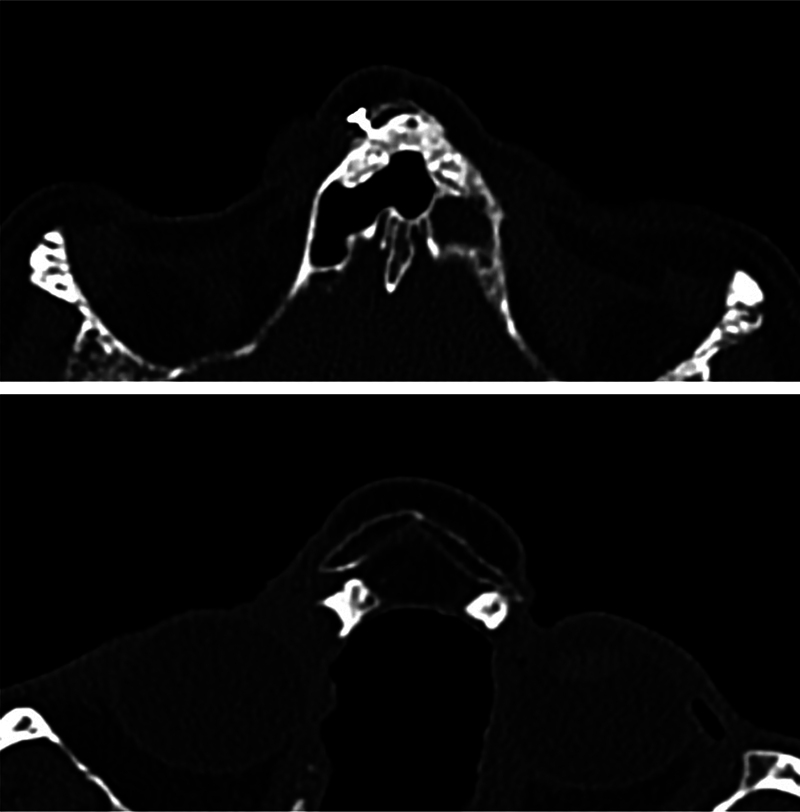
Axial computed tomography (CT) scan at 12 months of follow-up showing consolidation of the flap with the nasal spine of the frontal bone and right maxilla.
